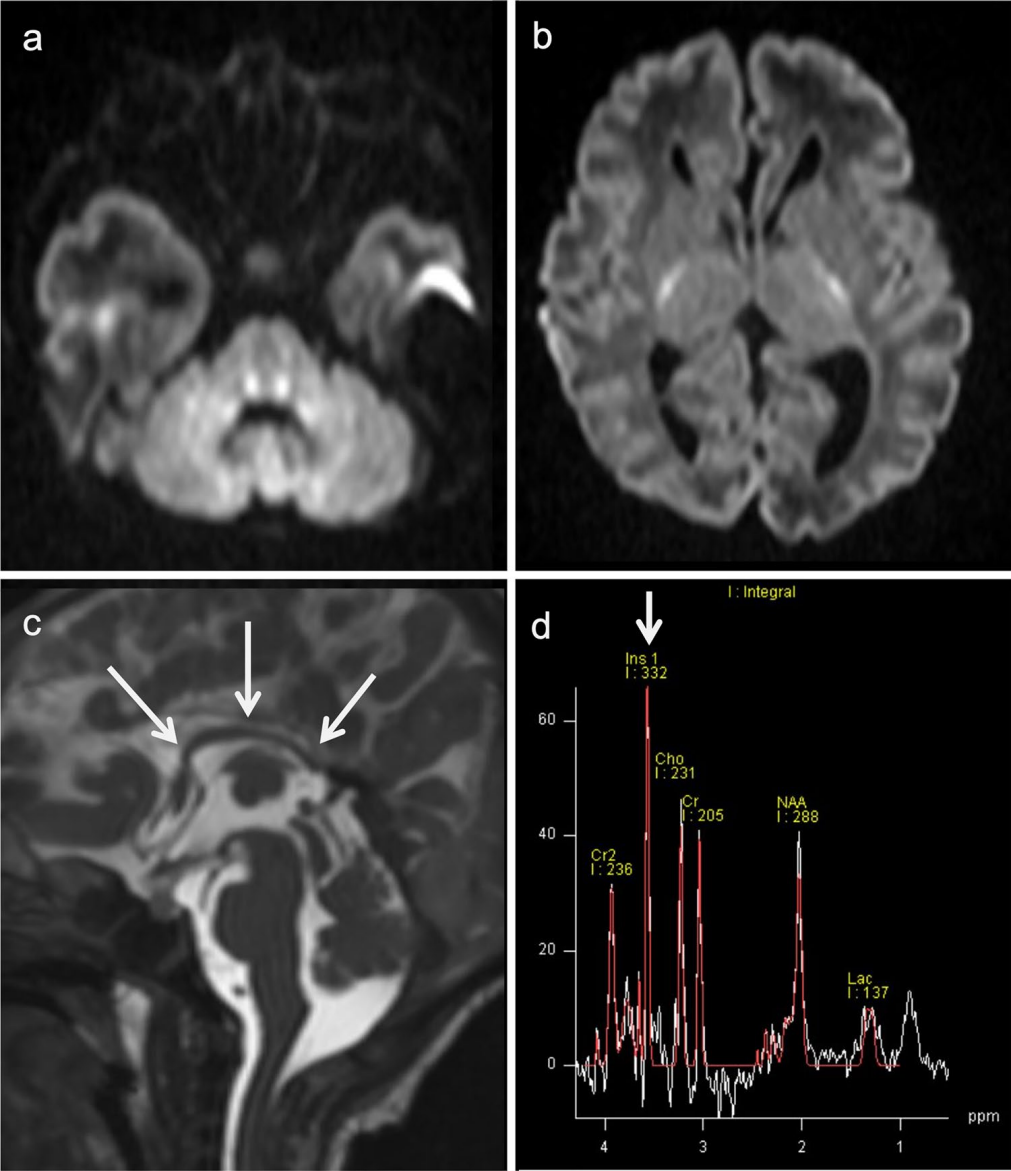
A 6-day-old neonate with nonketotic hyperglycinemia (NKH). a, b DWI demonstrated symmetrical high signals along the tegmental tracts and posterior limb of the internal capsules. c The corpus callosum was small and thin (arrows). d. ^1^H-MRS (TE = 30 ms) demonstrated a prominent, high, glycine and mIns peak at 3.55 ppm (arrow), which persisted on long TE MRS (TE = 270) (not shown) (Source: reference 5)
